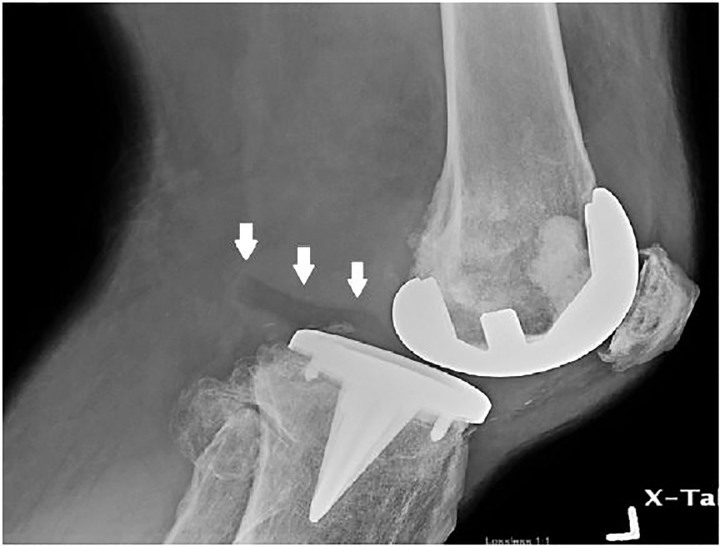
Lateral radiograph of the left knee showing posterior subluxation of the tibia with posterior displacement of the tibial polyethylene spacer (arrows).
